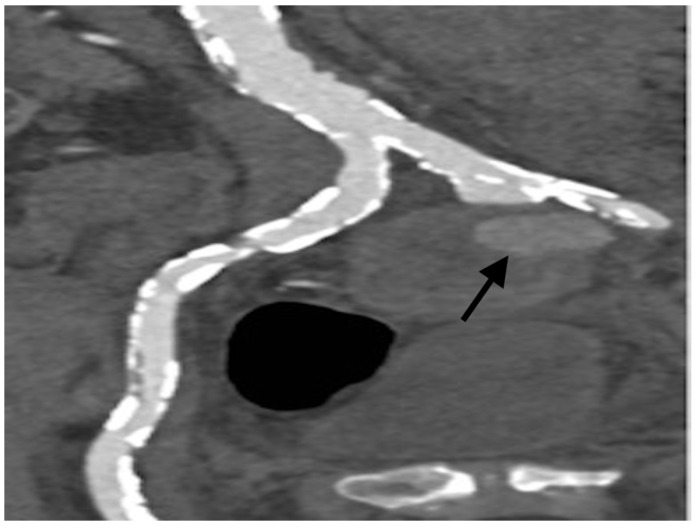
Coronal CTA with contrast demonstrating a PSA (black arrow) arising from the left internal iliac artery.
